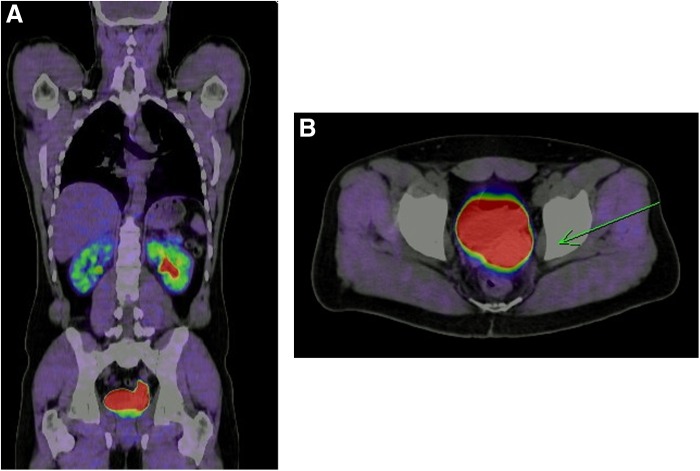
(A) Sagittal plane (18)F-DOPA-PET/CT of norepinephrin-producing tumor localized in the left seminal vesicle. (B) Transversal plane (18)F-DOPA-PET/CT of norepinephrin-producing tumor localized in the left seminal vesicle.
